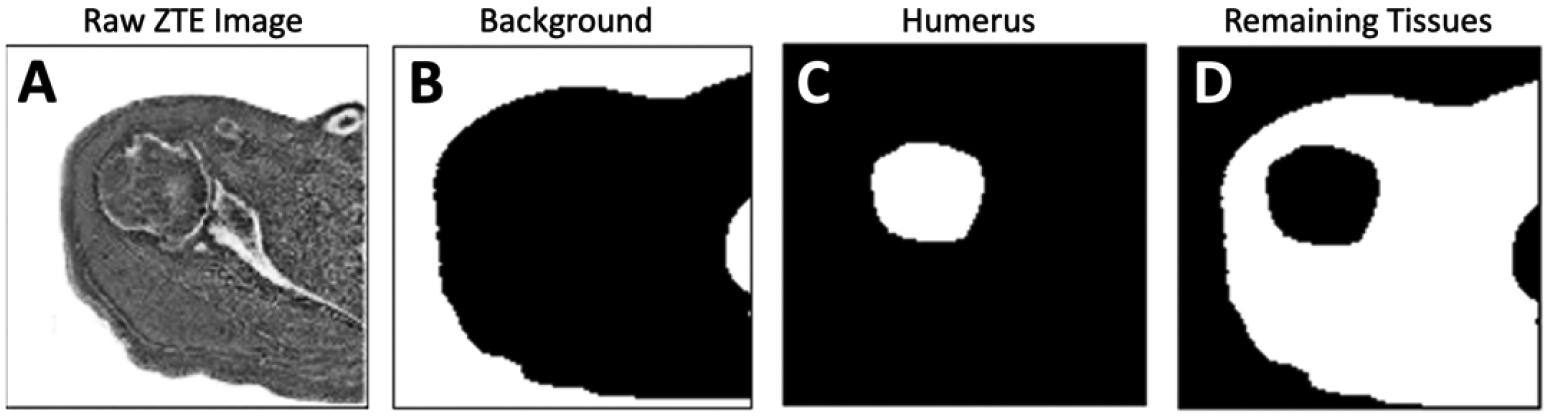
Manual segmentation of the MRI images. (A) Inverted ZTE MRI shoulder images acquired in the axial plane were manually annotated (segmented) into (B) background, (C) humeral head / humerus, and (D) the remaining tissues.
